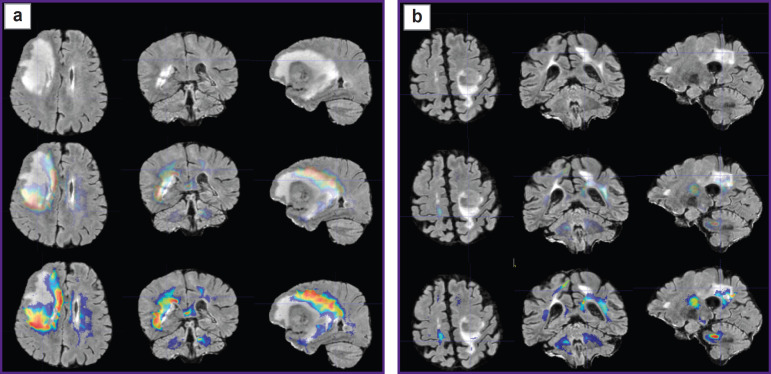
Plotting the maps of value gradients of the axonal water fraction (AWF) in patient with glioblastoma (а) and patient with metastasis (b) Upper row —T2-FLAIR images in three projections; middle row — comparison of T2-FLAIR with AWF parametric map (semitransparent for better contour visualization of the changed MR signal by T2-FLAIR); lower row — comparison of T2- FLAIR with AWF parametric map (in full color, where red and yellow tones show the zone of greatest parameter values)
